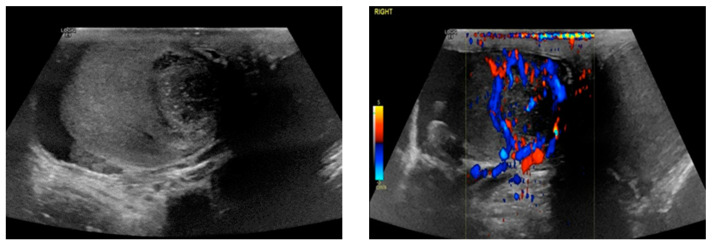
Biopsy-proven Testicular EP, case from our institution. Testicular ultrasound with B-mode and color Doppler shows a heterogeneous, hypoechoic lesion with punctuate calcifications and peripheral vascularity.
